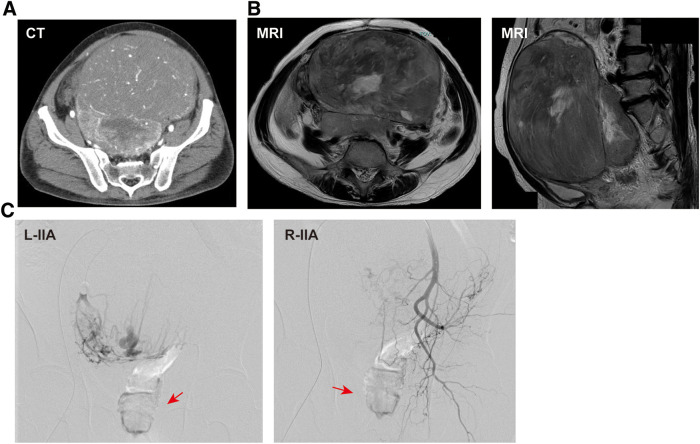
Preoperative imaging findings. (A) CT showing a 200 × 176 × 140 mm pelvic mass with intratumoral vessels and necrosis. (B) T2-weighted MRI demonstrating heterogeneous hyperintensity. (C) Angiography showing tumor hypervascularity with major feeders from the bilateral internal iliac arteries. The tumor is indicated by red arrows. IIA, internal iliac arteries; L, left; R, right
